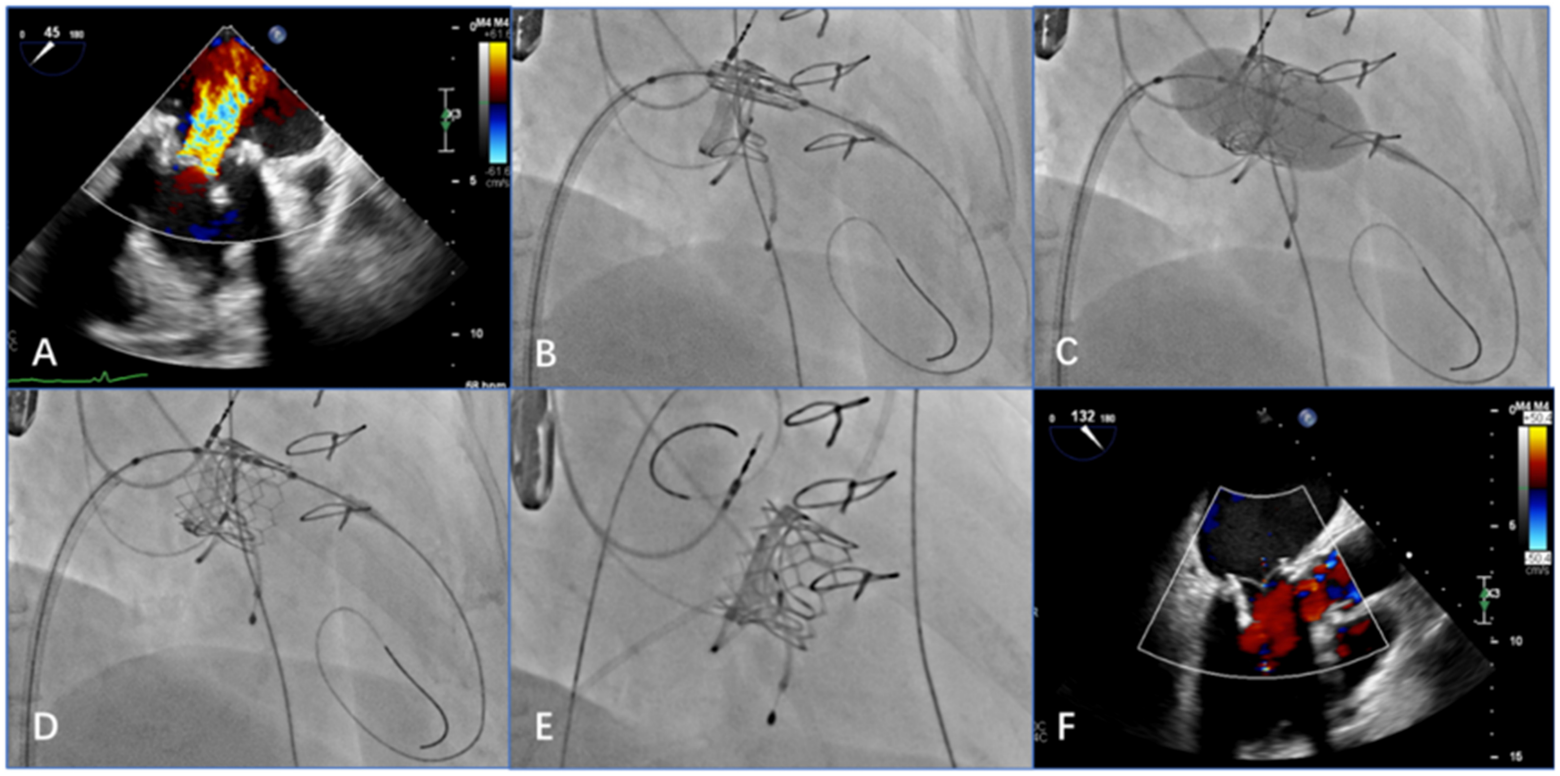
Transesophageal echocardiography (TEE) and digital subtraction angiography images show that the procedure achieved good results (e.g., data from patient 2). (A) Preprocedural TEE displays a large amount of colorful blood flow at the mitral valve. (B) The delivery system is positioned in relation to the mitral valve via the atrial septum. (C) After adjusting the position and the coaxiality, the balloon-expandable valve is inflated. (D) After expansion, the stent is fully unfolded. (E) When the guide wire is withdrawn, digital subtraction angiography shows that the position and shape of the mitral valve are ideal and that the stent fits closely to the valve. (F) Post-procedural TEE shows that the balloon-expandable valve is properly closed, with no paravalvular regurgitation.
